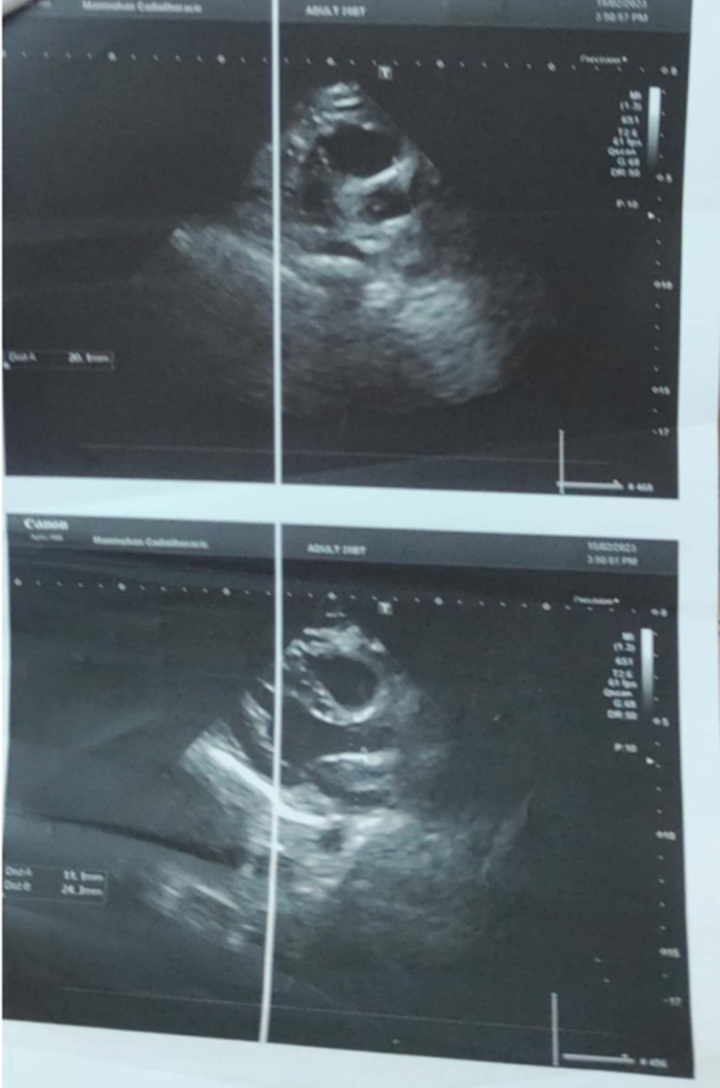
Ultrasonography showing features of chronic kidney disease.
